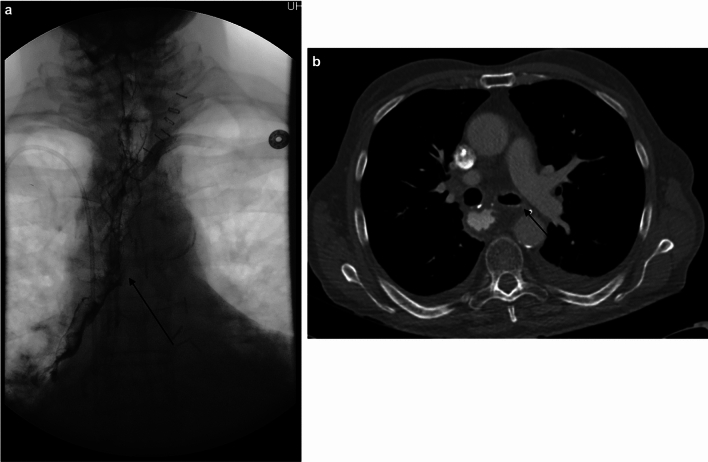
Esophagogram (a) demonstrates a tracheoesophageal fistula (arrow) with contrast opacification into the bilateral bronchial tree. Axial CT (b) shows contrast pooling in the esophagus with small amounts of contrast in the dependent aspect of the left mainstem bronchus (arrow)
